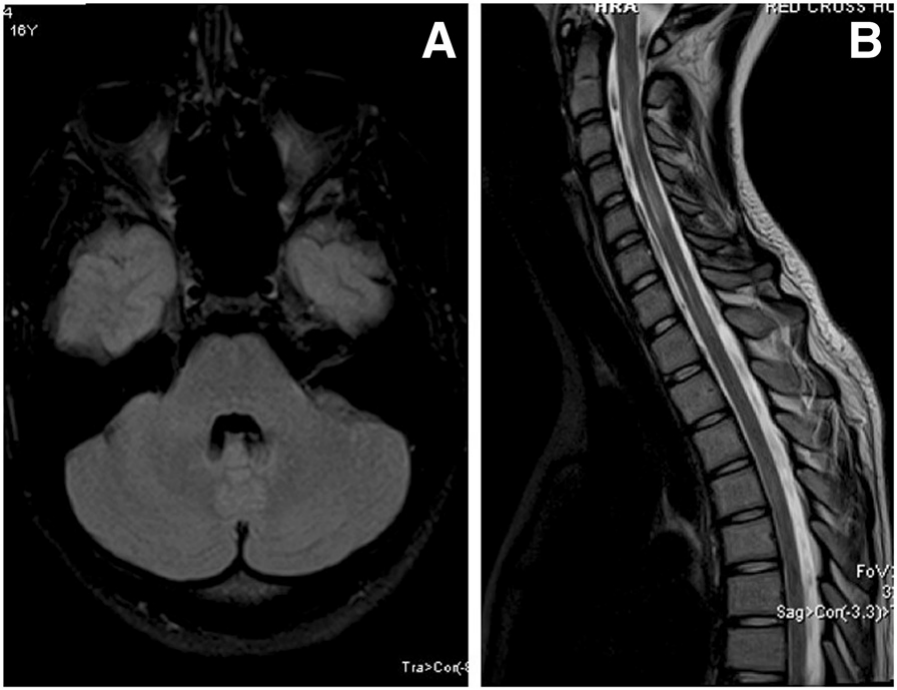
A. MRI Flair axial of the brain; normal. B: T2 sagital cervical and upper thoracic spine; intramedullary lesions of cervical and thoracic spine C3 –Th5.
